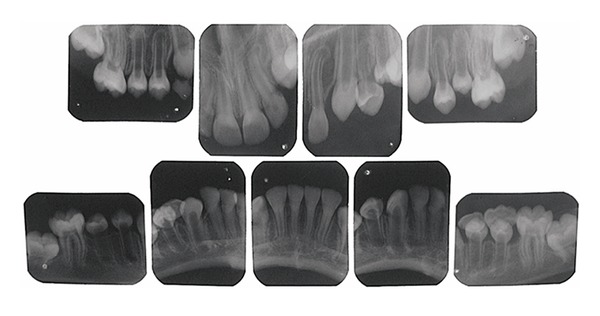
Periapical X-ray showing taurodontic teeth with enlarged pulp chambers and insidious early obliteration of the coronary pulp chambers of the lower incisors.
